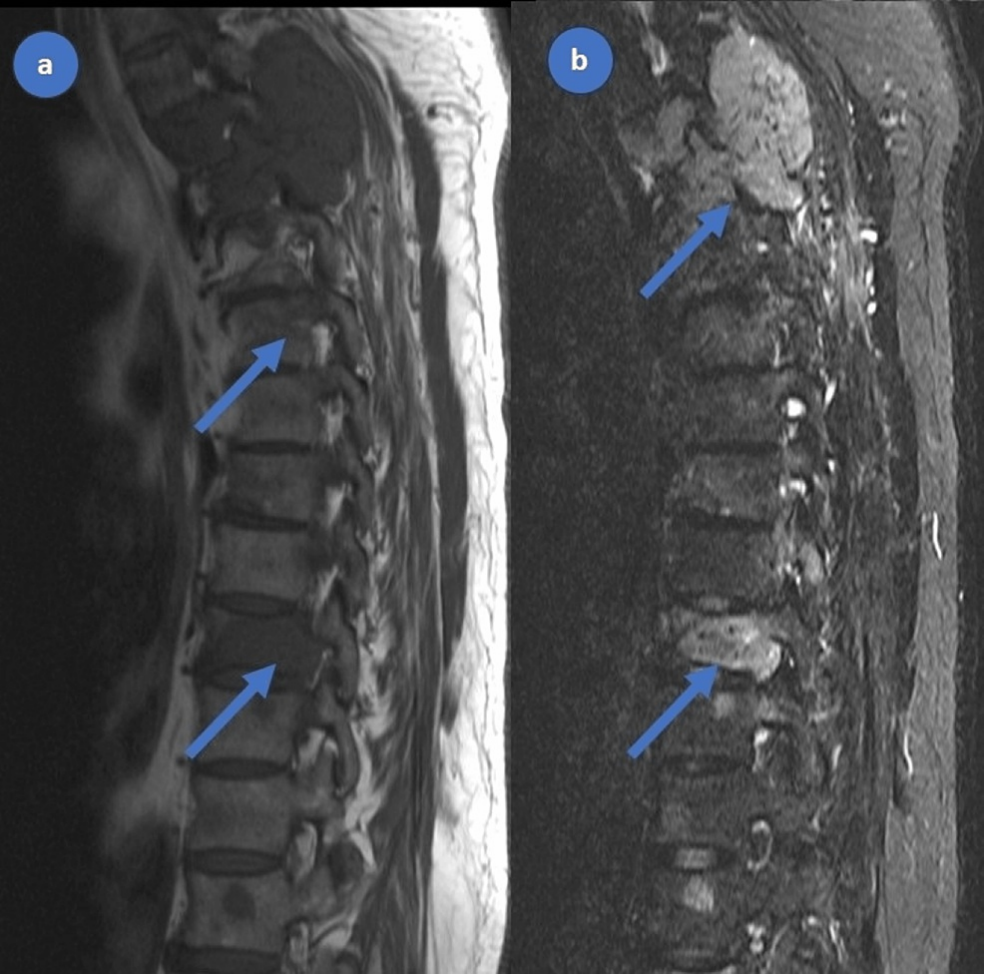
MRI spine demonstrating multiple lesions of the spine, involving vertebral bodies, posterior elements, and paraspinal soft tissue components (a, b)
